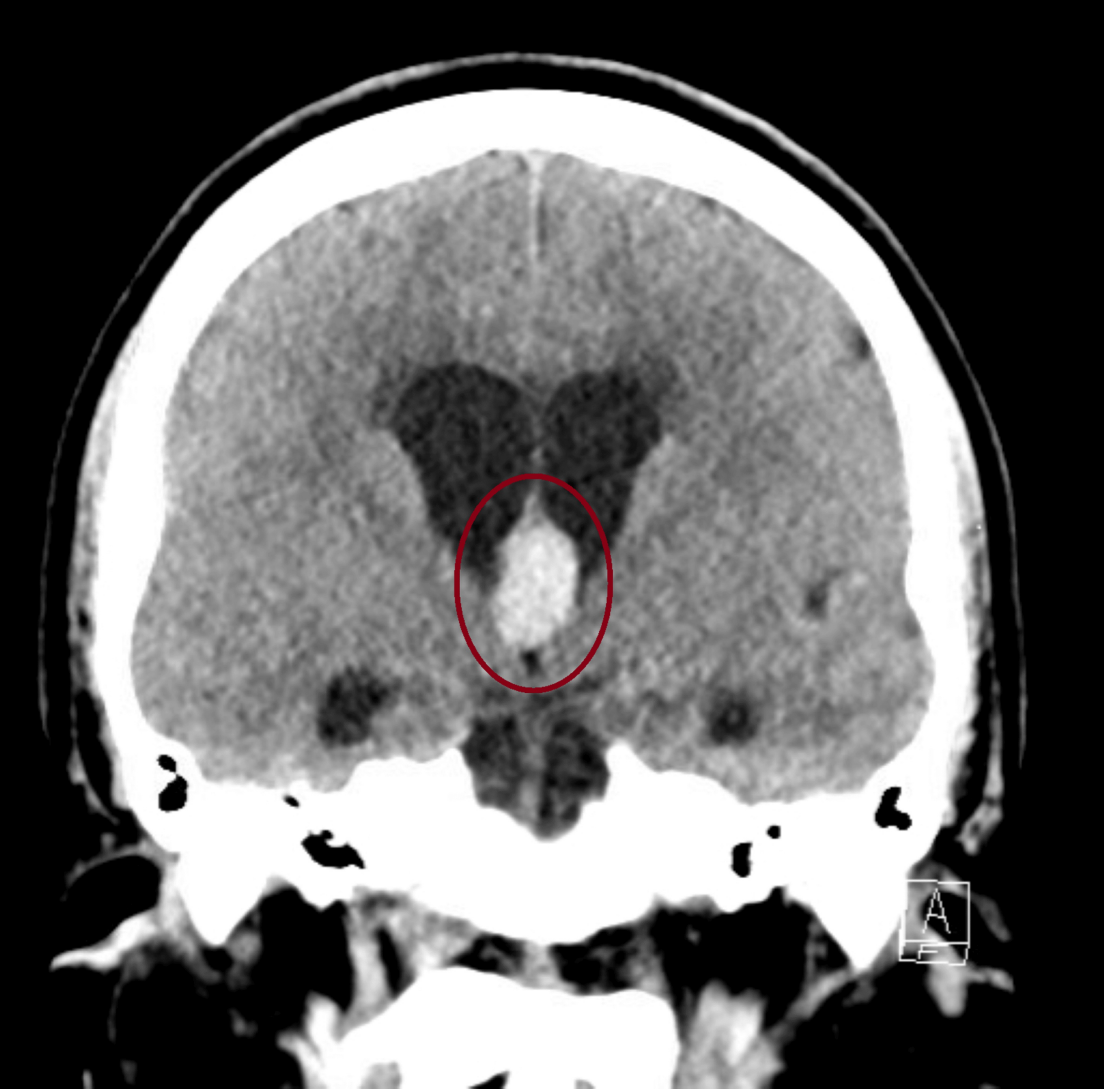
Axial non-contrast CT showing a hyperdense colloid cyst at the foramen of Monro with associated ventricular enlargement.
